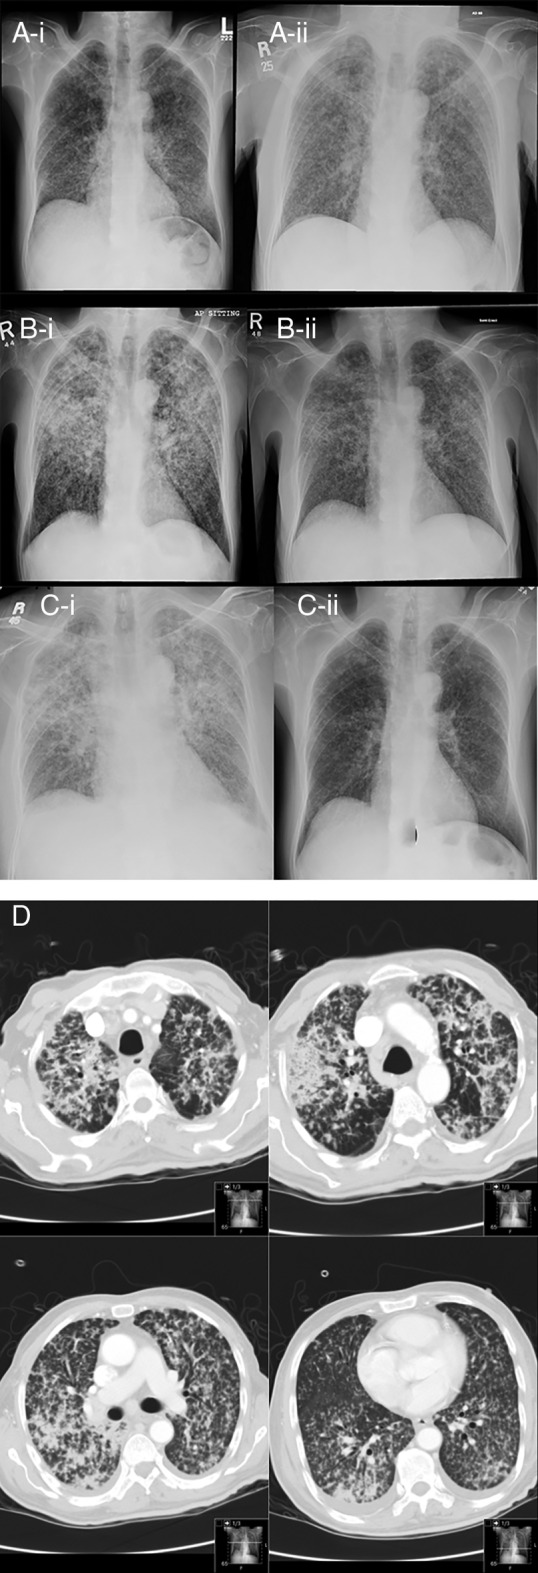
Chest radiographs on the first (A), second (B), and third admissions (C) (i, on admission; ii, on discharge). (D) Selected images of computed tomography (CT) of the chest during the second admission.
